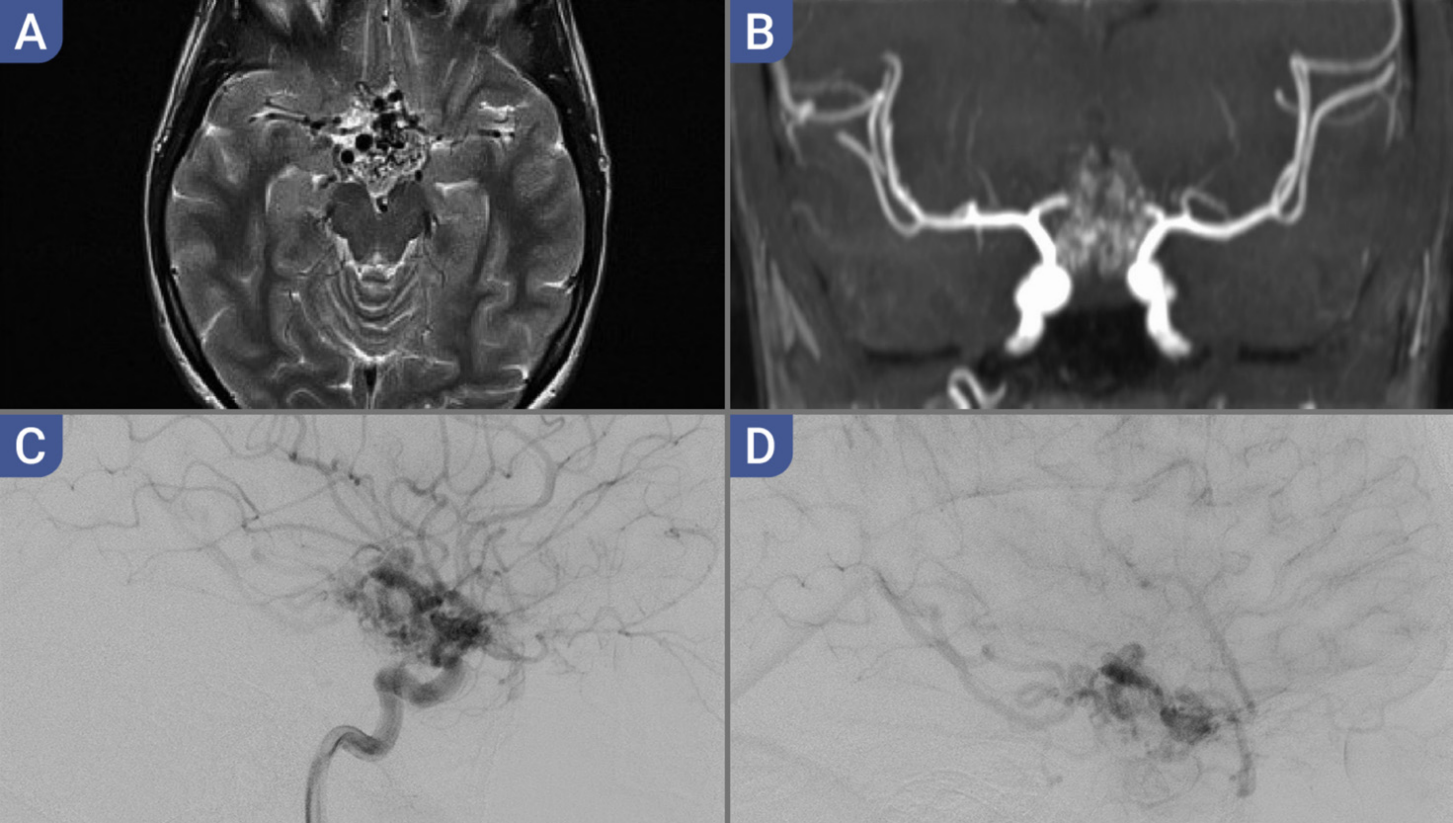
(A) Magnetic resonance imaging (MRI) of the brain with angiography 1.5T T2 transversal. (B) MR angiography of the brain 1.5T 3D time-of-flight maximum intensity projection reconstruction. (C) Digital subtraction angiography latero-lateral projection of the right internal carotid artery (ICA) arterial phase. (D) Digital subtraction angiography right ICA injection latero-lateral venous phase.
